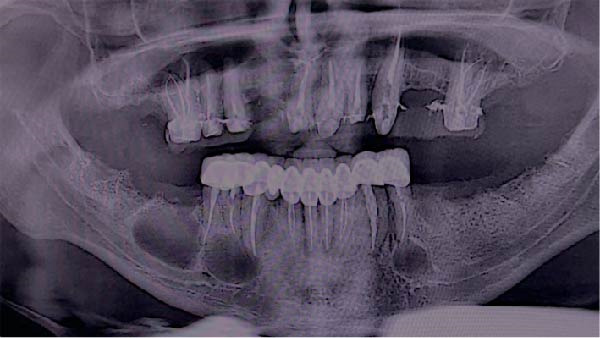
Preoperative panoramic radiograph showing bilateral well‐defined radiolucent lesions with radiopaque margins.
